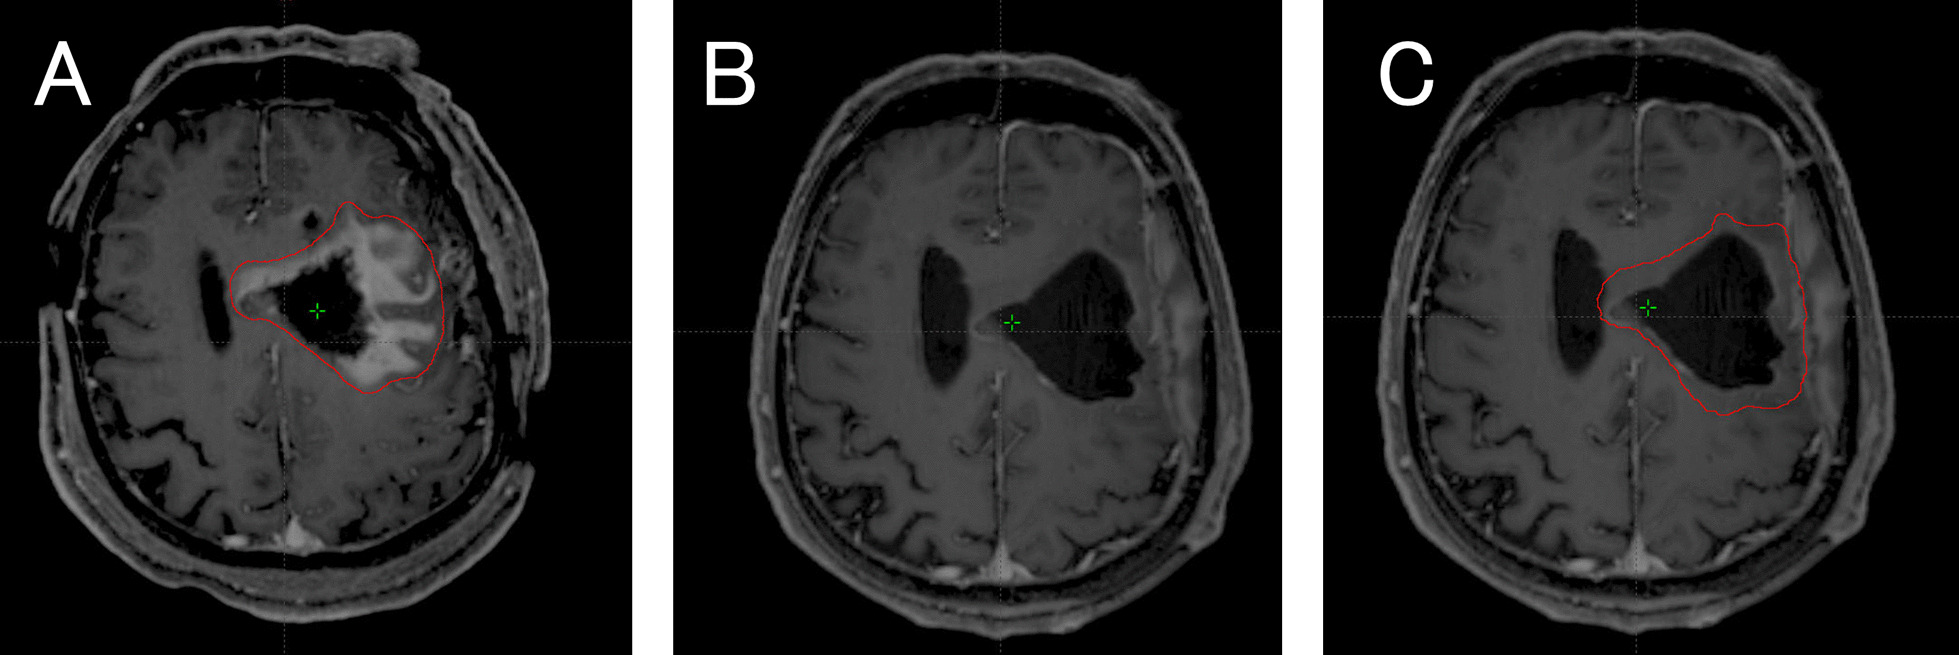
Contouring procedures in ART. A Boost GTV (GTV-pre) in postoperative contrast-enhanced T1 weighted MRI (MRIpre). B The contrast enhancement area was decreased in contrast-enhanced MRI during radiotherapy (MRImid). C Boost GTV (GTV-mid) in MRImid. ART, adaptive radiation therapy; GTV, gross target volume; MRI, magnetic resonance imaging
